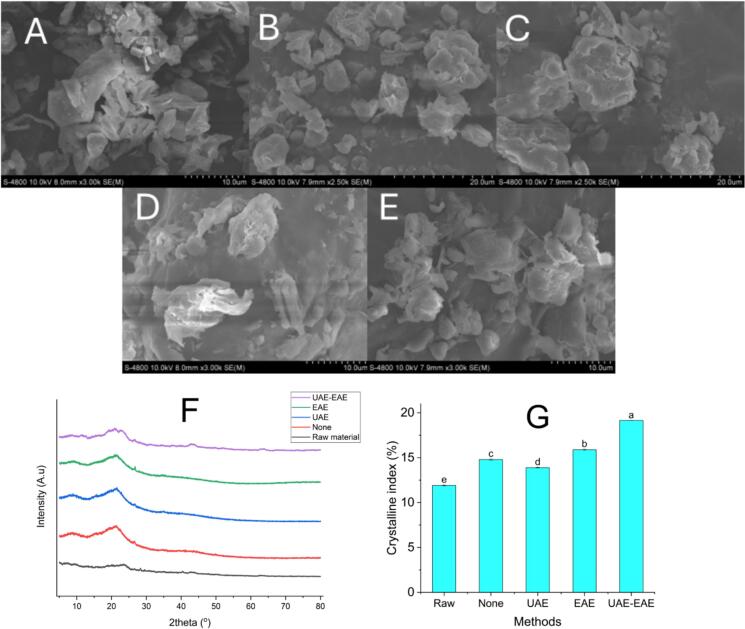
Comparative analysis of surface morphology and X-ray diffraction patterns between untreated and NADES-treated ECLP; (A): SEM image of raw ECLP; (B): SEM image of untreated ECLP; (C): SEM image of ECLP treated with UAE; (D): SEM image of ECLP treated with EAE; (E): SEM image of ECLP treated with UAE-EAE; (F): X-ray diffraction of ECLP from 5 methods; (G): Cellulose crystallinity index (CCI) values of untreated and NADES-treated ECLP samples (Different letters (a, b, c, d, e) indicate statistically significant differences). UAE: ultrasonic-assisted extraction technique; EAE: enzymatic-assisted extraction technique; UAE-EAE: ultrasonic-enzymatic-assisted extraction technique.
